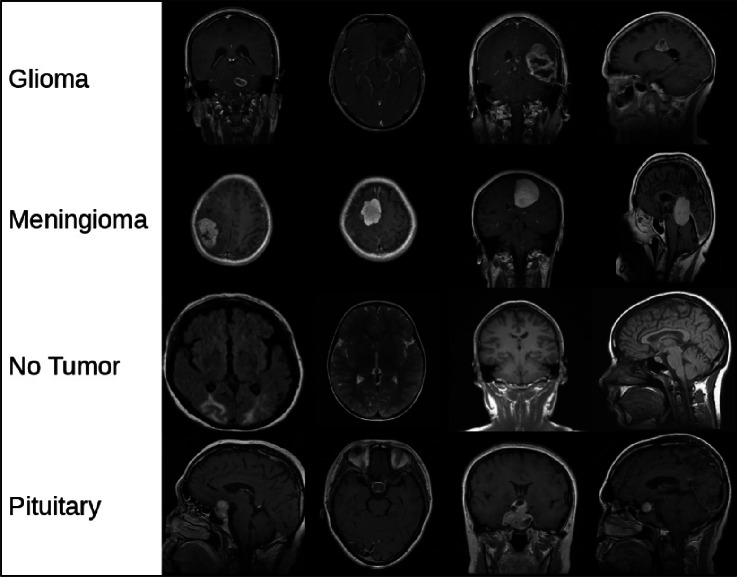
Representative MRI samples from the dataset. Each row shows the three anatomical views (axial, coronal, sagittal) for one tumor class. From top to bottom: glioma, meningioma, no tumor (healthy), and pituitary tumor. The dataset exhibits significant variability in tumor appearance, size, and contrast across different views and classes.
